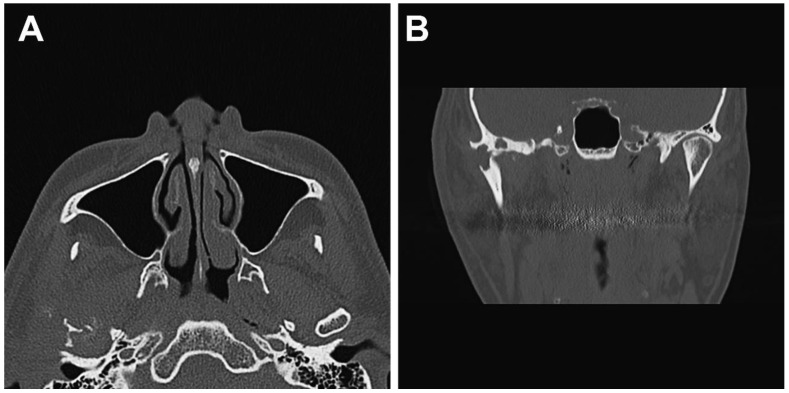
Postoperative computed tomography (CT) images. (A) Axial CT images and (B) Coronal CT images revealed that the masses were extracted from the right temporomandibular joint.
